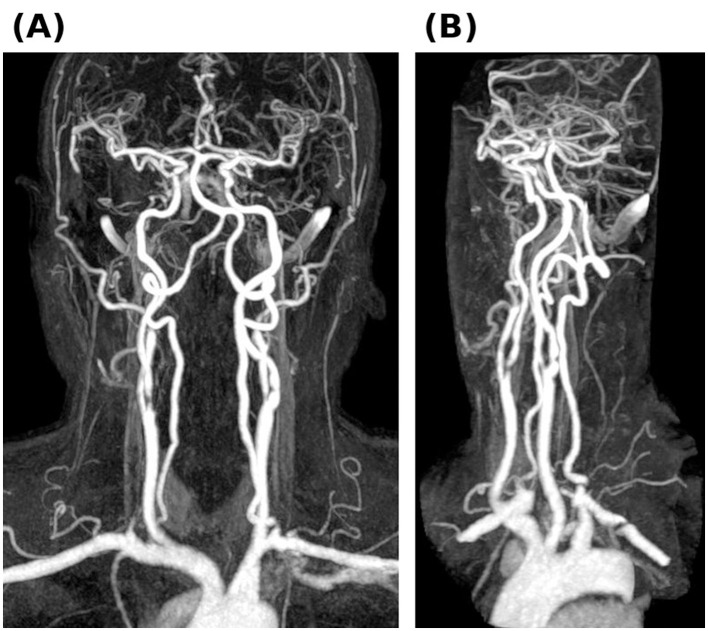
Contrast-enhanced magnetic resonance angiography (CE-MRA, T1-weighted sequences) of the same patient as in Figure 1, demonstrating 70%−99% stenosis (NASCET) of the right internal carotid artery (RICA). (A) Coronal maximum-intensity projection (MIP) of the cervical and intracranial arteries. (B) Oblique sagittal MIP of the right cervical carotid system. CE-MRA enables simultaneous assessment of the supra-aortic vessels and the intracranial circulation without ionizing radiation, and supports the identification of plaque vulnerability features when combined with high-resolution vessel-wall imaging. Source: authors' personal archive.
